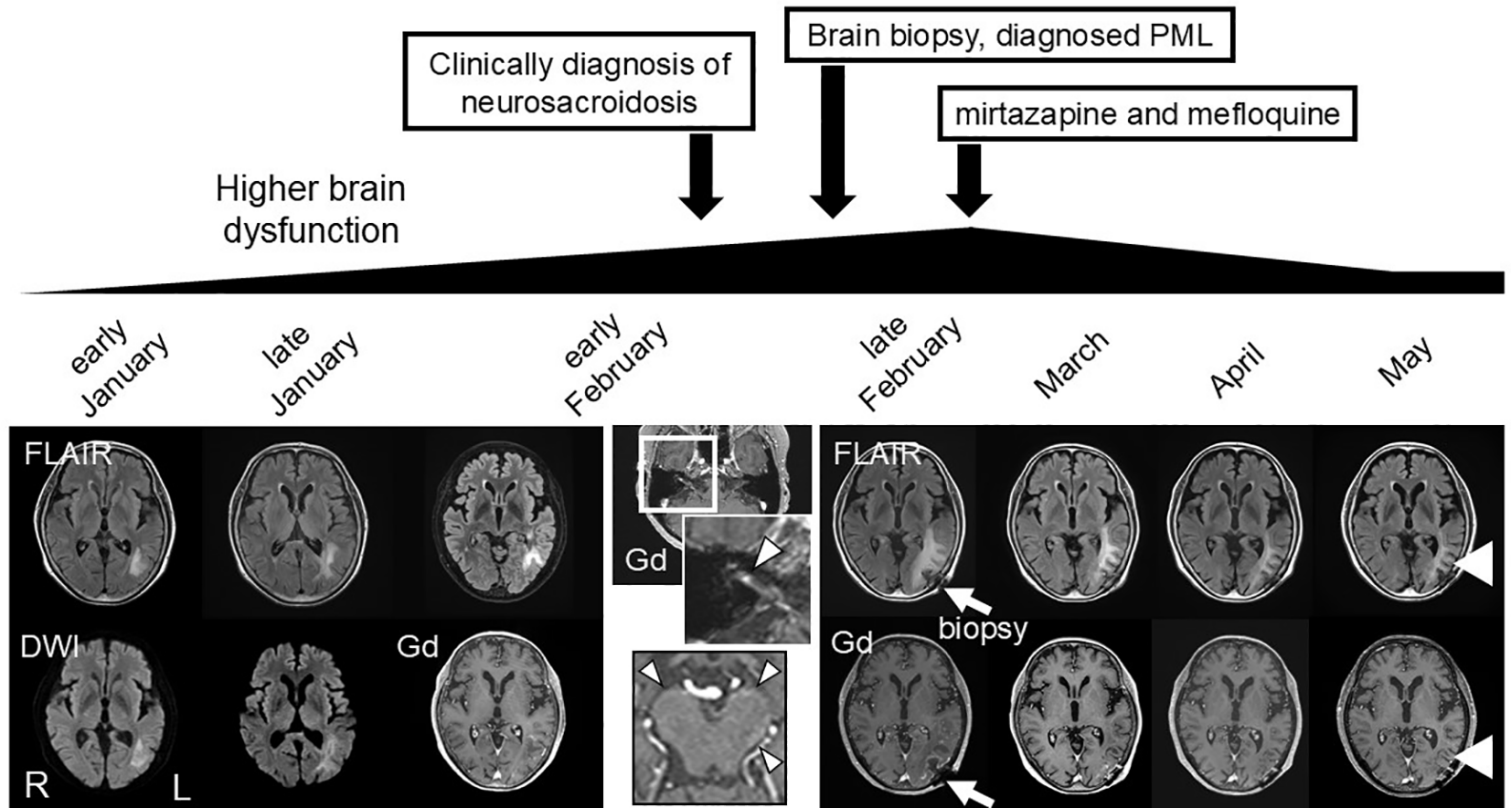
Clinical course and MRI findings. Magnetic resonance imaging (MRI) findings. Diffusion-weighted imaging (DWI) and fluid attenuated inversion recovery (FLAIR) revealed an enlarging hyperintense lesion in the left temporal, occipital, and parietal lobes before treatment. Gadolinium-enhanced T1-weighted images revealed an enhancing area in the lesion, and enhancement of the right cranial nerve VII (arrowhead), and leptomeninges in the brainstem (arrowhead). Brain biopsy was performed for the enhancing brain parenchymal lesion in late February (arrow), and mirtazapine and mefloquine treatment was started in March. Higher brain dysfunction and the MRI lesion gradually improved following the treatment (arrowhead).
